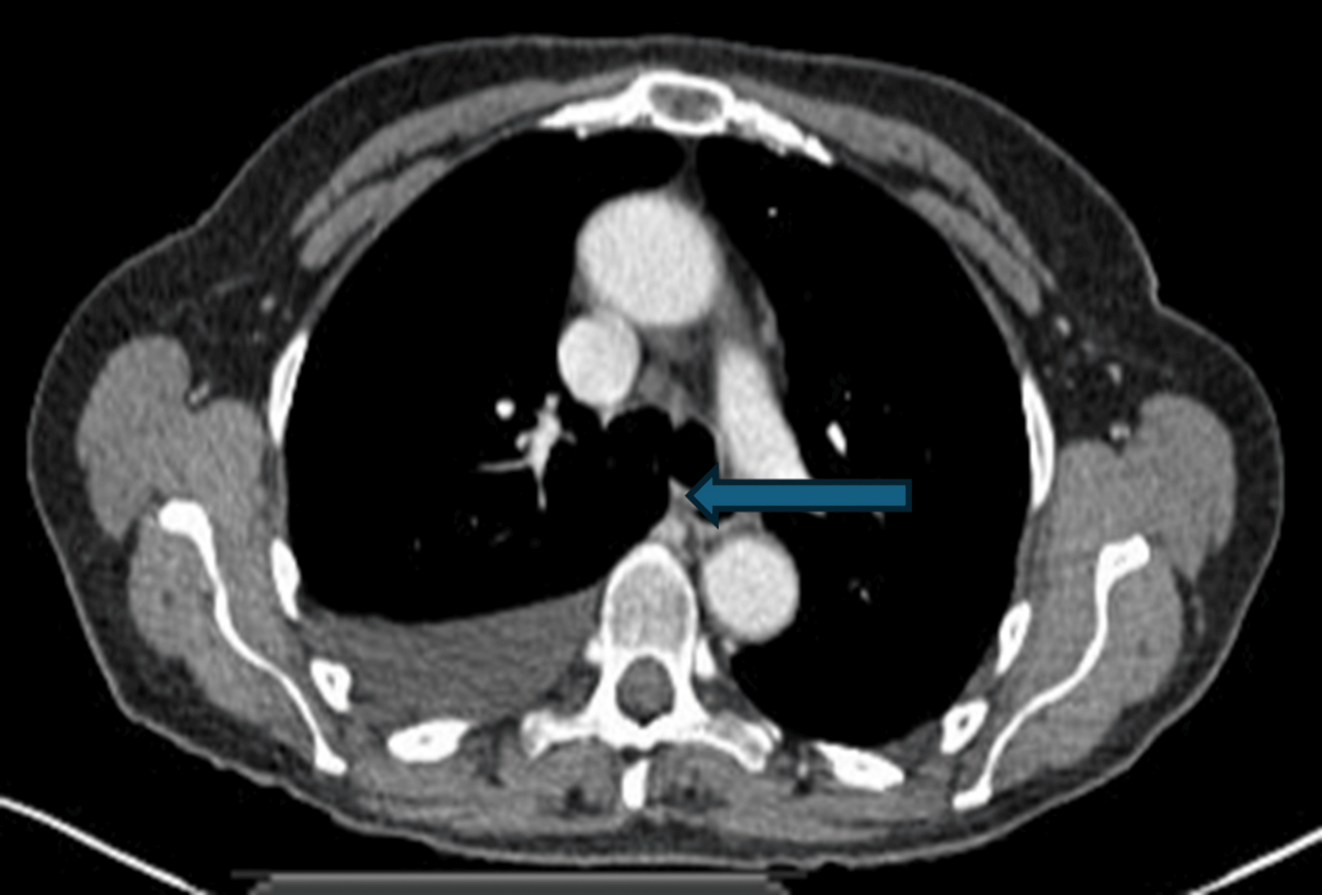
CT thorax axial section showing a pre-tracheal lymph node measuring 1.7 cm (blue arrow)
